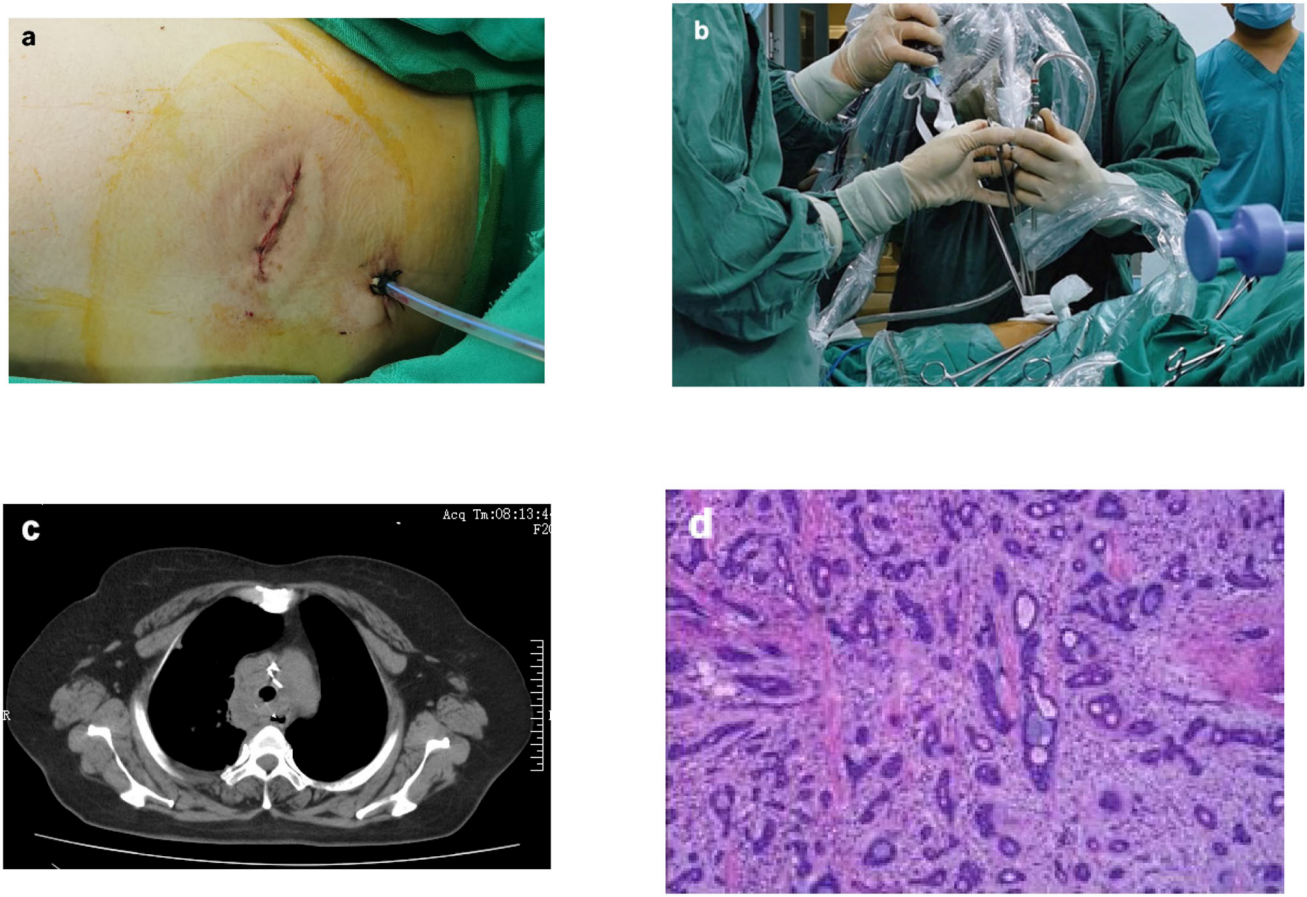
Surgical photos and postoperative examination of the patient. The surgical incision was ~4 cm (a); The endoscope and surgical instruments were introduced via the same incision (b); Postoperative CT revealed that the trachea was patent (c); Postoperative pathologica results revealed adenoid cystic carcinoma (ACC) (d).
